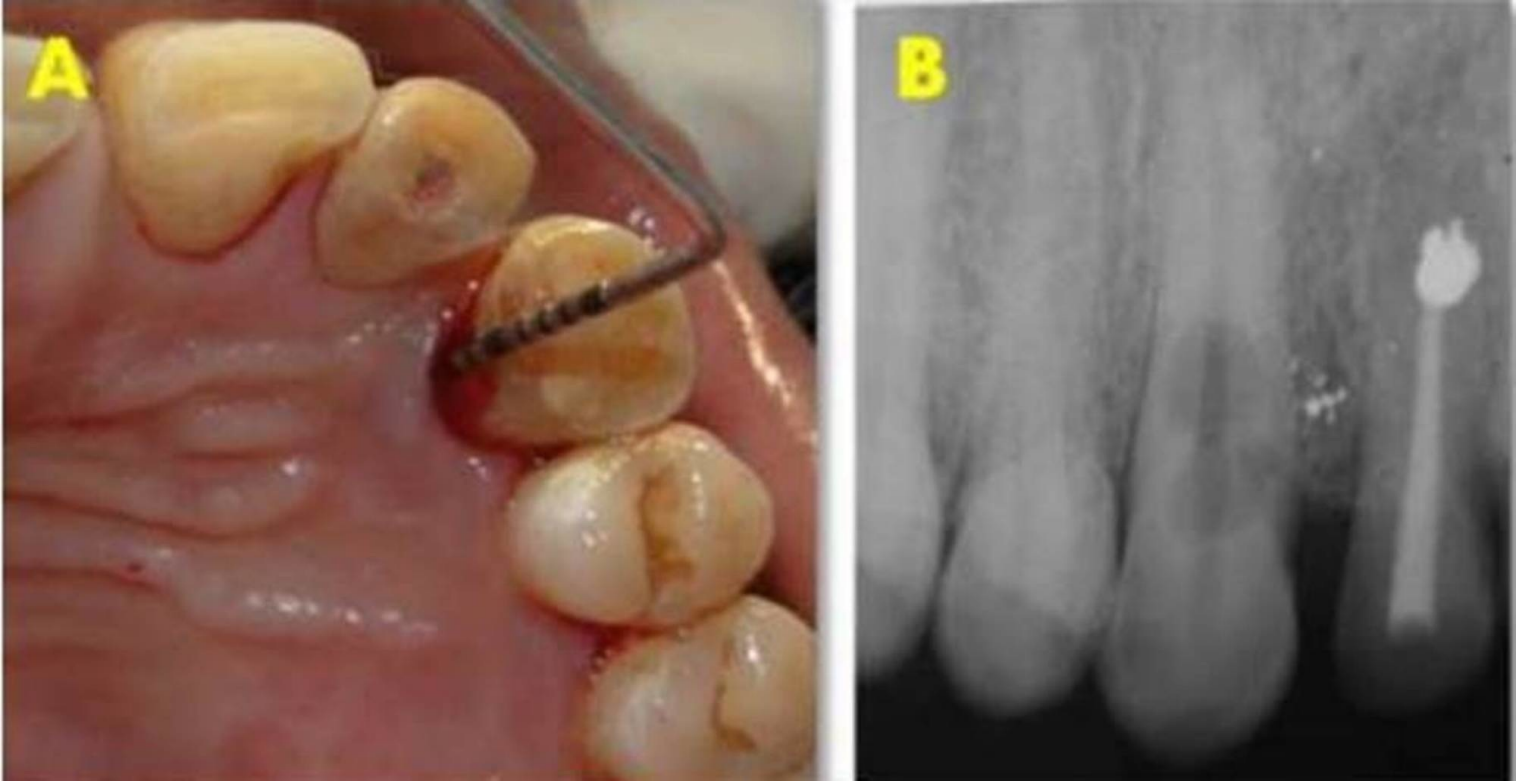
Preoperative clinical and radiographic assessment. (A) Deep periodontal pocket in tooth 23 present lingually. (B) Preoperative radiograph.
